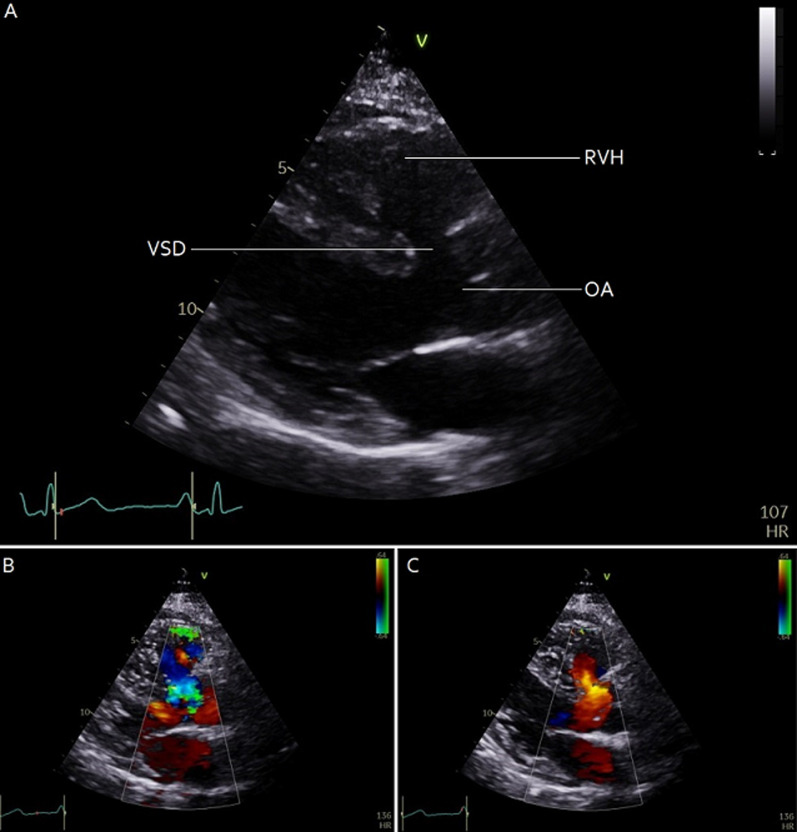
A) 2D echocardiogram in parasternal long axis view demonstrating features of tetralogy of Fallot, including ventricular septal defect (VSD), right ventricular hypertrophy (RVH) and overriding aorta (OA); B) colour Doppler demonstrating right-to-left shunt through the VSD during systole; C) and left-to-right shunt during diastole
